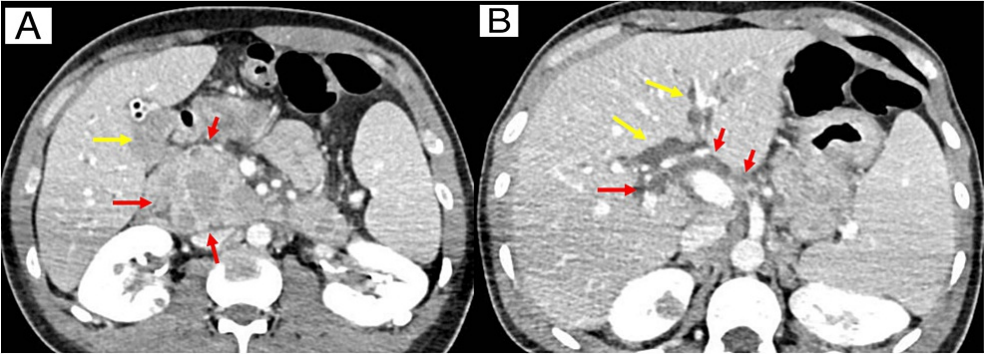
Abdominal CT scan images (axial view) (A) The image shows dilation of the bile ducts (yellow arrows), probably due to compression, which may correspond to granulomatous diseases (red arrows), such as disseminated tuberculosis or neoplastic dissemination of Burkitt's lymphoma. (B) The image shows multiple peripancreatic necrotic lymph node enlargements in the hepatic hilum (red arrows), exerting a compressive effect on the bile ducts.
